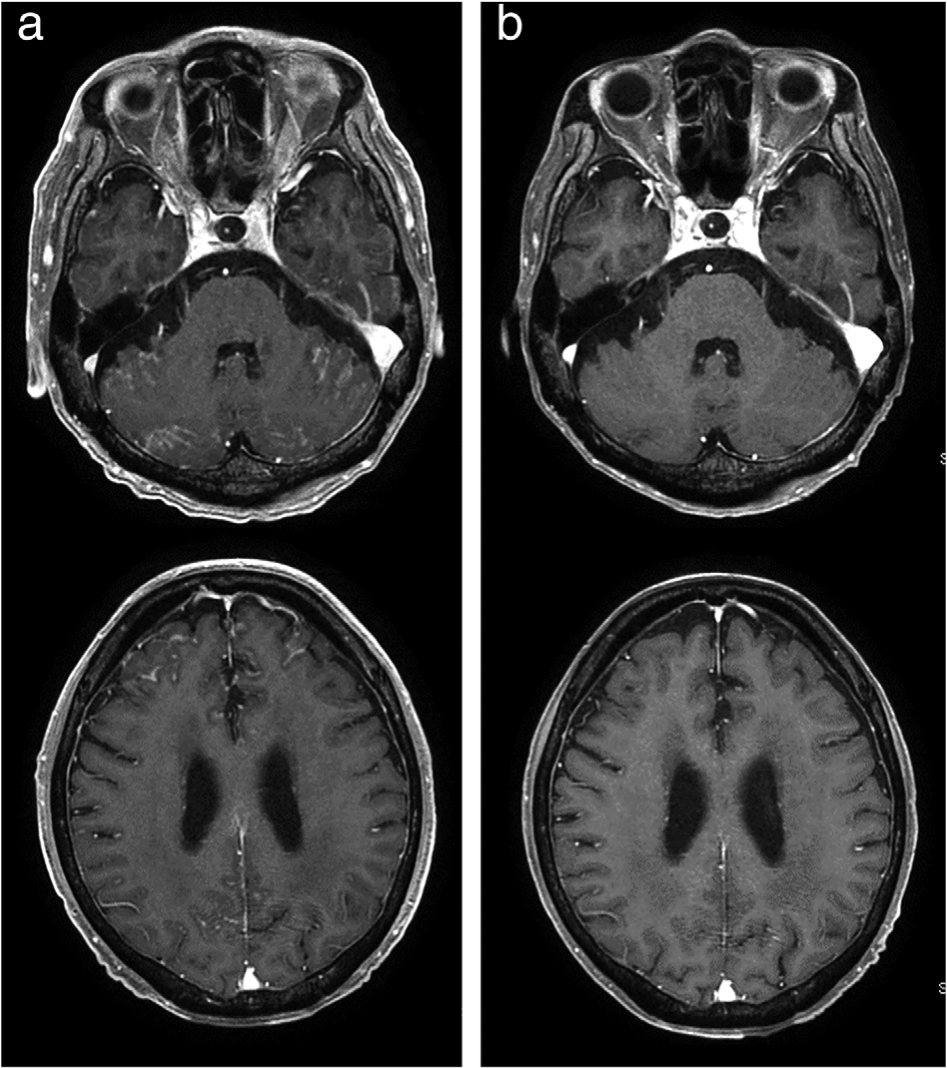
Brain magnetic resonance imaging showed (a) enhancement of the leptomeninges and small nodules of the cerebellar folia and frontal lobe, all of which diminished following treatment with dacomitinib (b).
